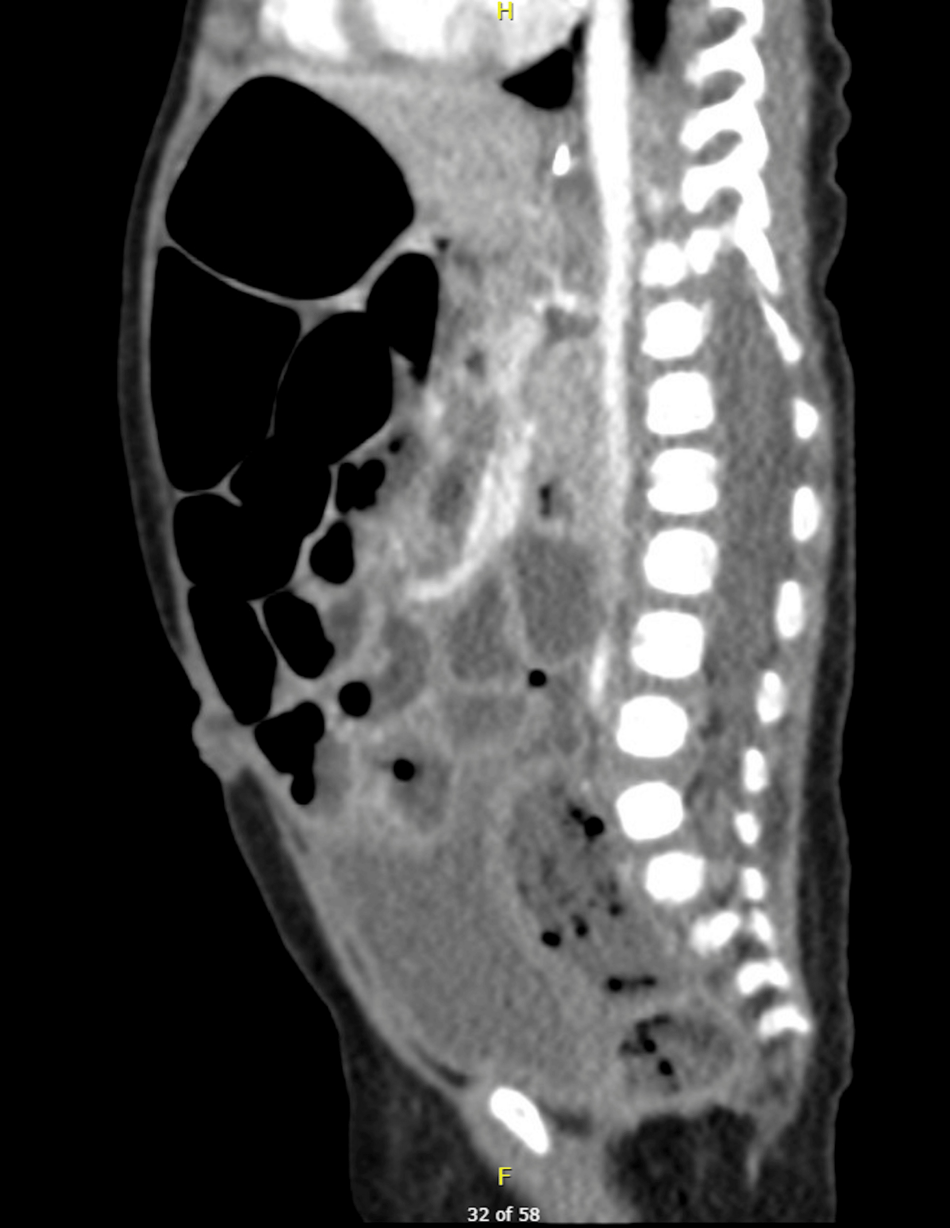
CT of the abdomen (lateral view). Sagittal views of the patient’s abdominal CT showing a distended bladder and stomach, with a feeding tube in situ. No evidence of pneumatosis intestinalis or pneumoperitoneum. No obvious transition zone or signs of malrotation.
